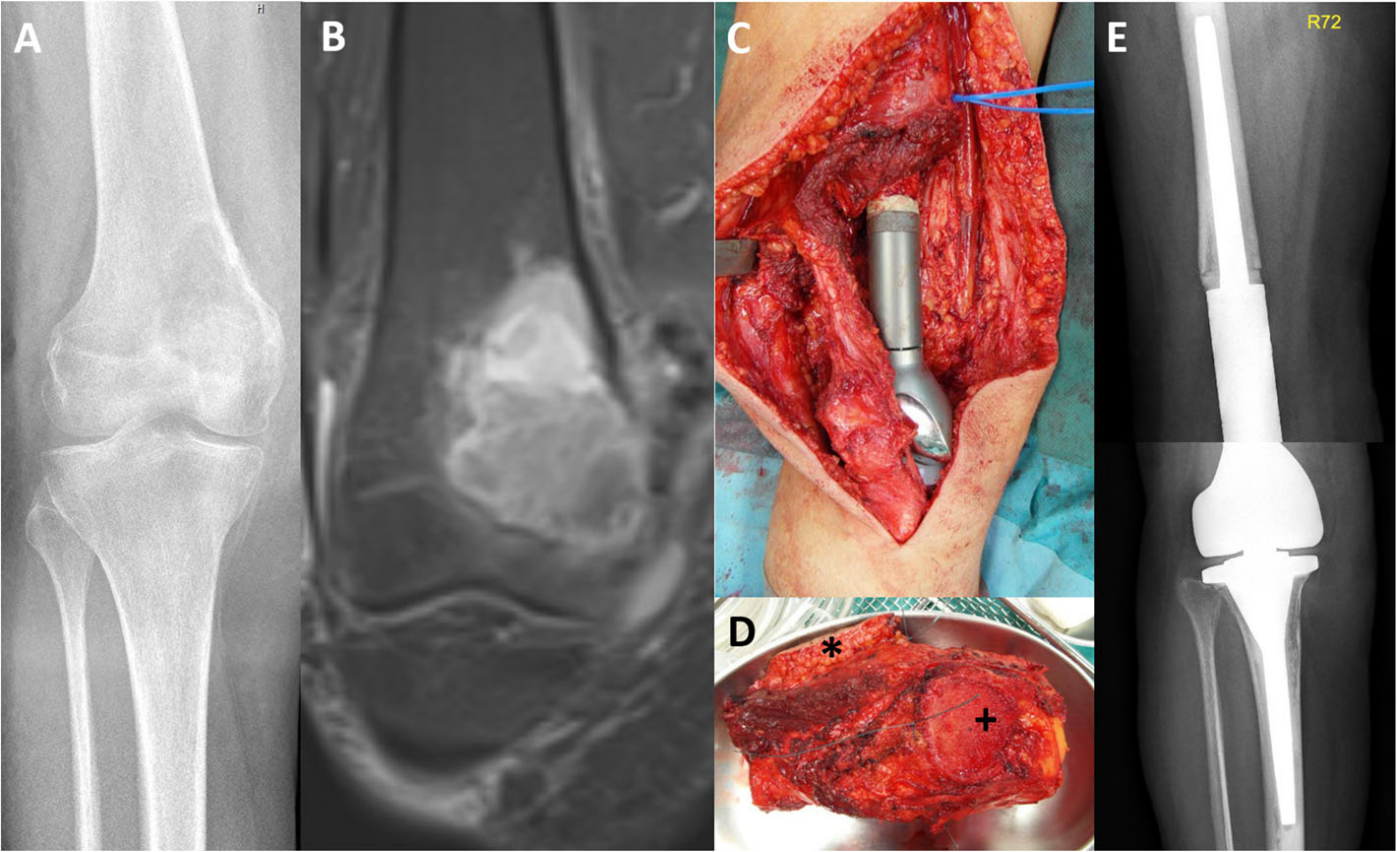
Case of a 75-year-old female patient with a Ewing-like sarcoma of the right distal femur with infiltration of the joint space. A Conventional radiography showing the osteolytic lesion. B Magnetic resonance imaging with contrast agent shows erosion of the articular cortex of the medial femur condyle. C Intraoperative photograph after extra-articular knee resection and implantation of a megaimplant. The patella was split in the coronal plane. D Resected knee (top: medial, left: proximal) with the articular part of the patella (*) and the biopsy scar (+) attached to it. E Postoperative antero-posterior radiograph
